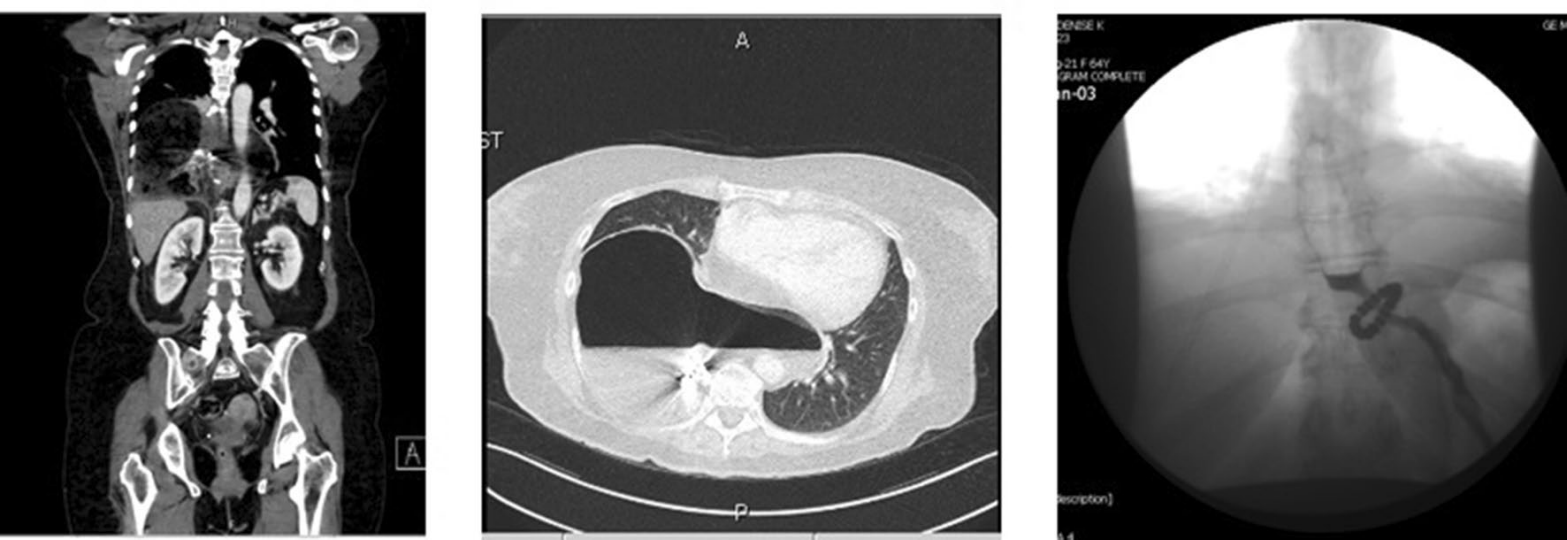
CT scan of a patient with type III recurrence. Patient underwent reoperation for repair of PEH and LINX device was left in position. The postoperative esophagram after reoperation demonstrates an appropriately positioned LINX® and no herniation
